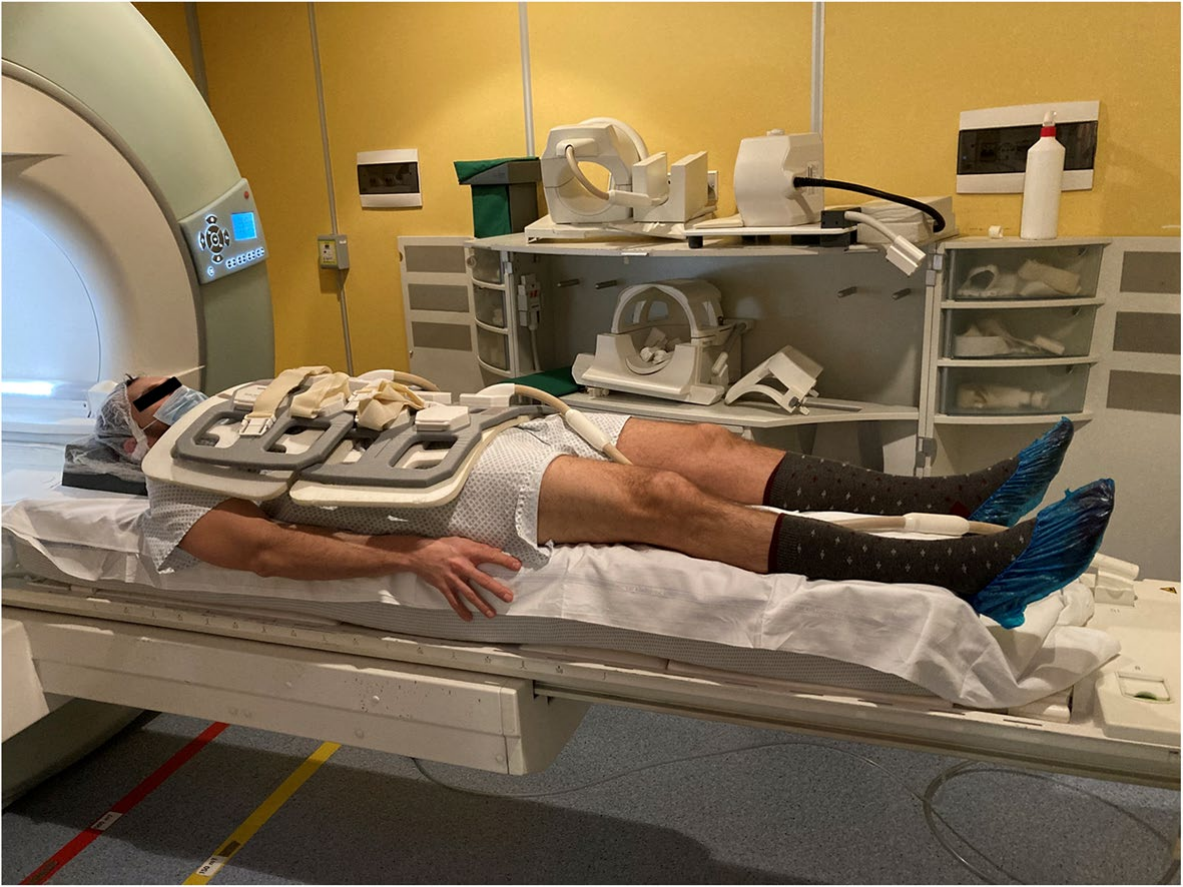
A study subject laying down on the mattress before MRI acquisition
